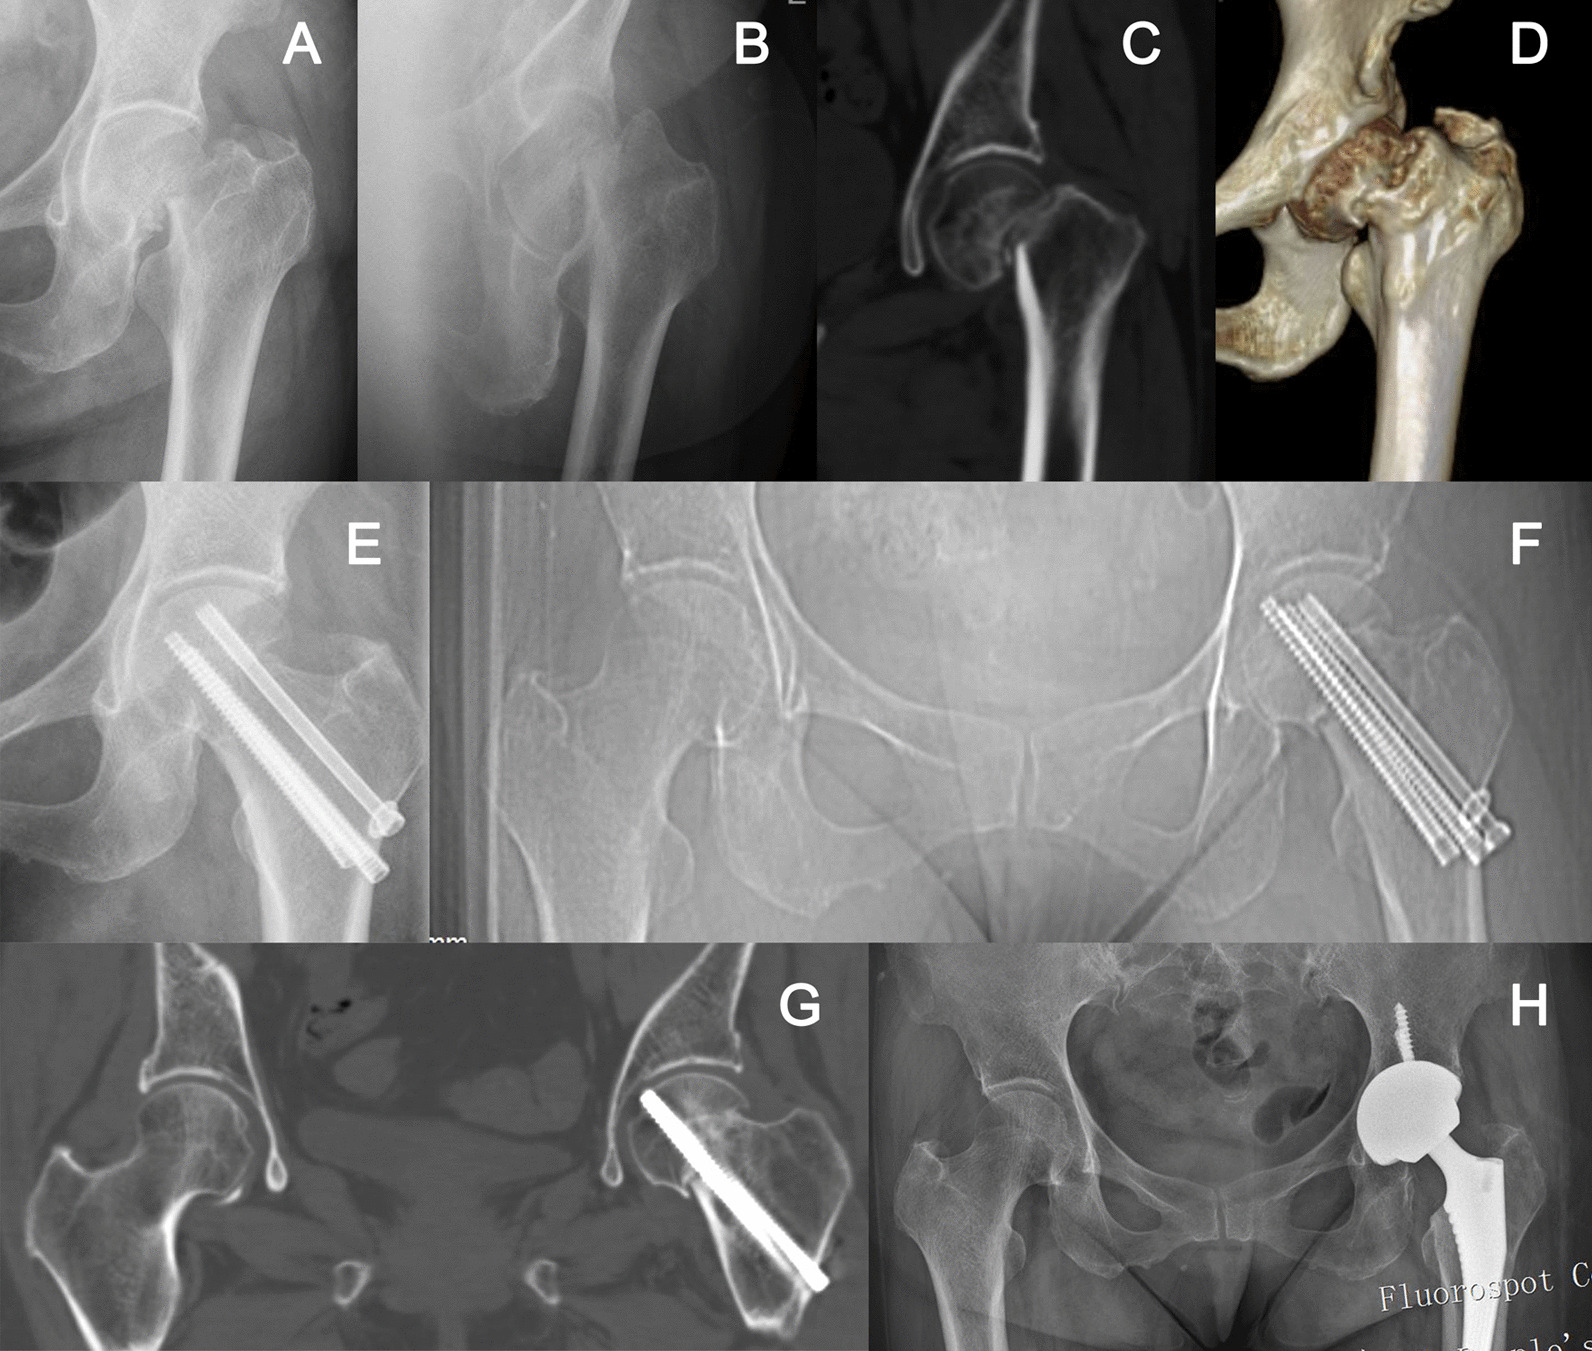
Radiographs of a 53-year-old female who sustained a FNF. A–D Anteroposterior (AP) X-ray radiographs and CT scan images of vertical displaced fracture, type Pauwels III and Garden IV; E AP radiograph following closed reduction and internal fixation with CBS; F–G AP radiograph and CT coronal reconstruction image revealing the nonunion with a distinctive fracture fixation loosening, medial screw migration beneath the femoral head subchondral bone in the direction of the acetabulum with slight fracture displacement; H The patient underwent a total hip arthroplasty due to the nonunion after 9 months following the primary internal fixation
